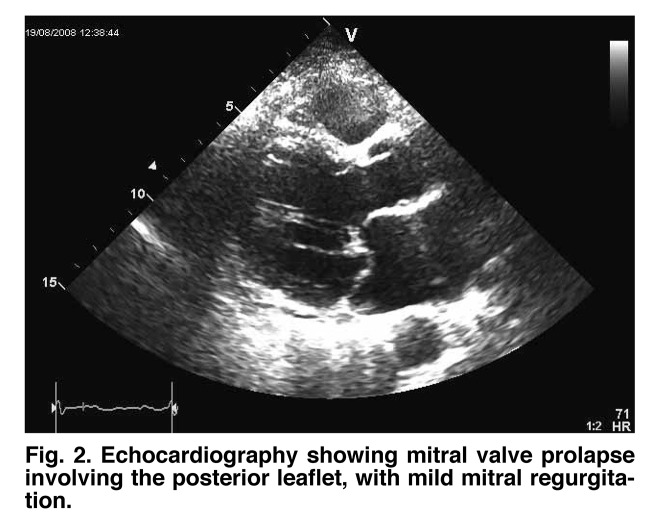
Echocardiography showing mitral valve prolapse involving the posterior leaflet, with mild mitral regurgitation.
